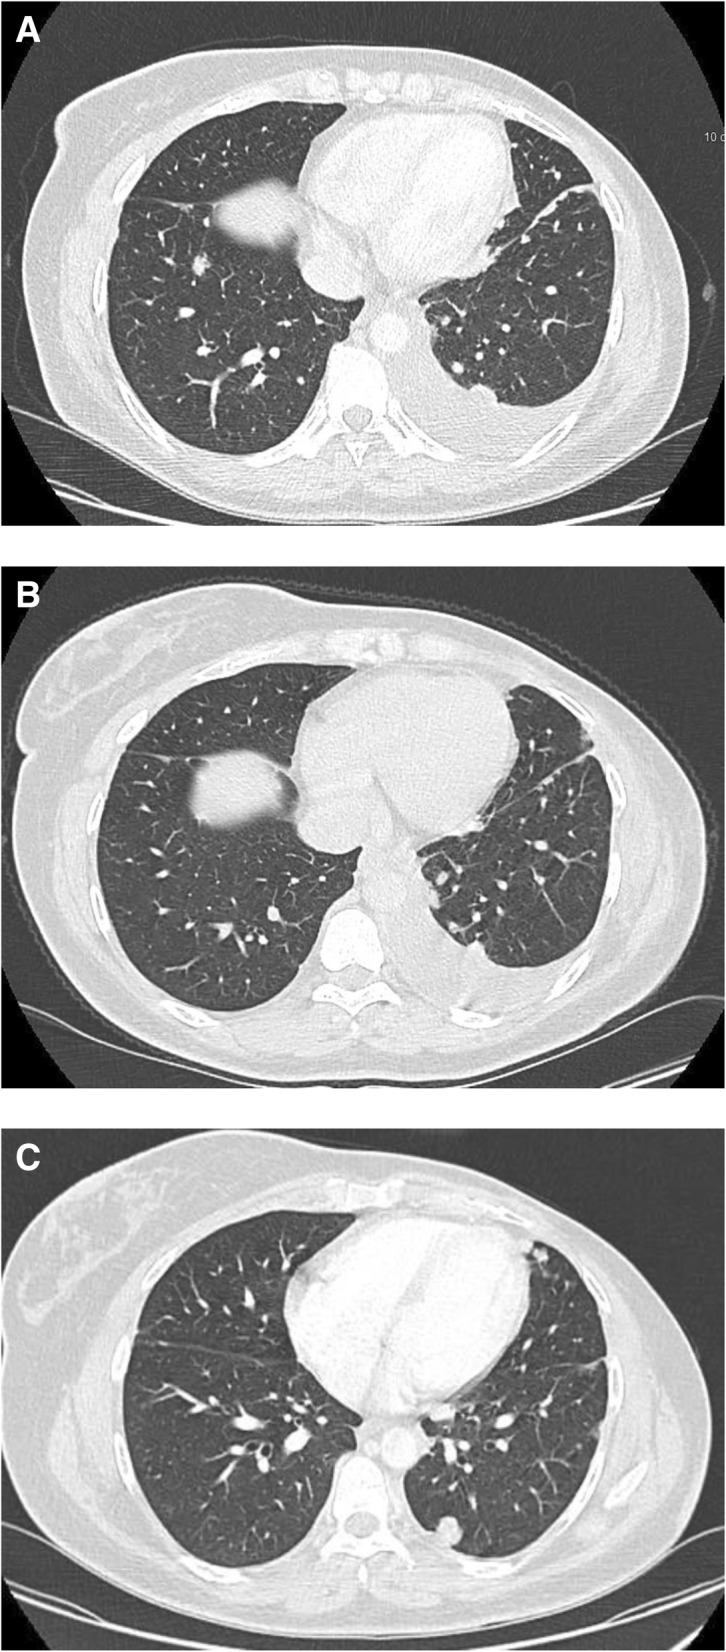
a CT scan showed irregular interlobular septal thickening and micronodular opacities. The infiltration and diffuse nodules distributed throughout the lymphatic vessels evocated lymphangitic carcinomatosis. b CT scan after the fourth cycle of the chemotherapy showed disappearance of micronodular invasion and a reduction of pulmonary nodules. c CT scan after 6 months with eribulin treatment
